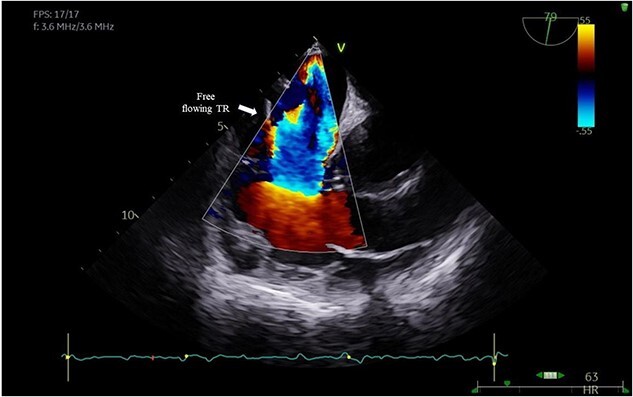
Colour Doppler showing free flowing TR.
